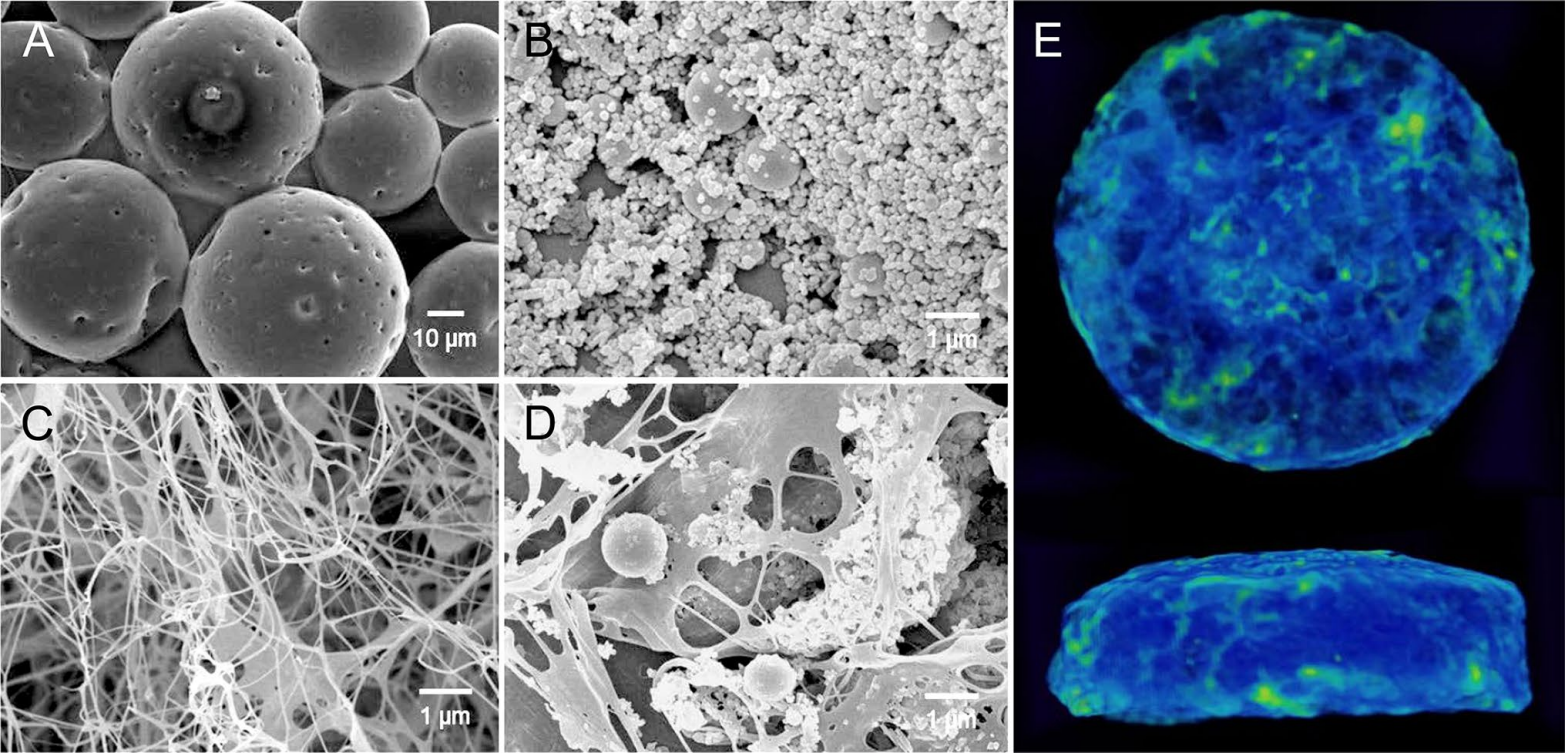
Morphological analysis of CHAS. SEM images of (A) PLGA microspheres; (B) hydroxyapatite; (C) collagen scaffold; (D) CHAS; (E) µ-CT images of CHAS (green threshold level at 147).
